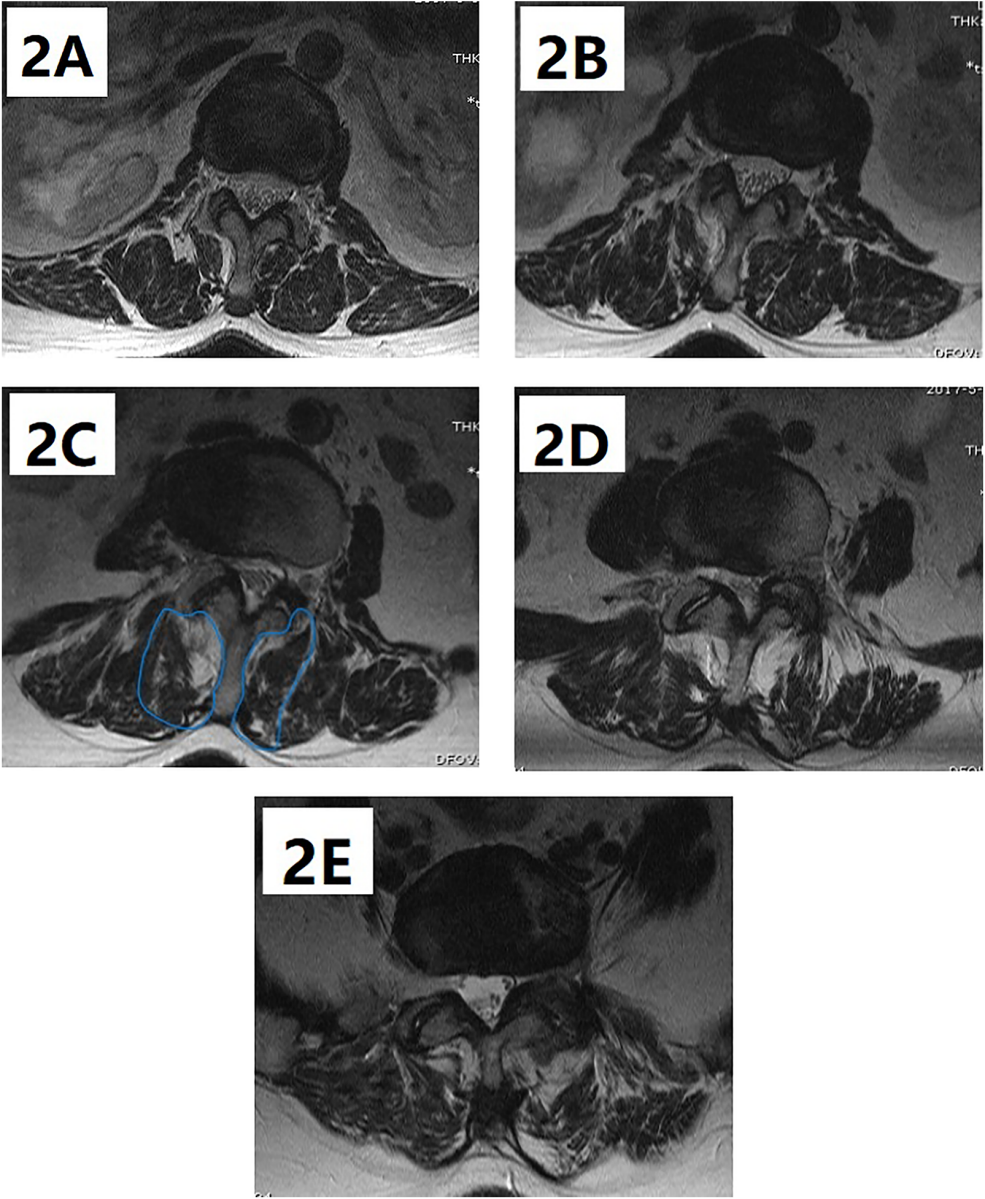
(A–E) Fat tissue on the MRI is colored in white; FIA% of the multifidus muscle on the convex side is 27.09%, 14.26%, 30.37%, 33.36%, and 30.69%, and on the concave side, it is 40.67%, 42.77%, 39.25%, 34.16%, and 43.02% at the L1-2, L2-3, L3-4, L4-5, and L5-S1 levels, respectively; the asymmetric degree of the multifidus muscle is 12.82% and the average degree of the multifidus muscle is 33.57%.
